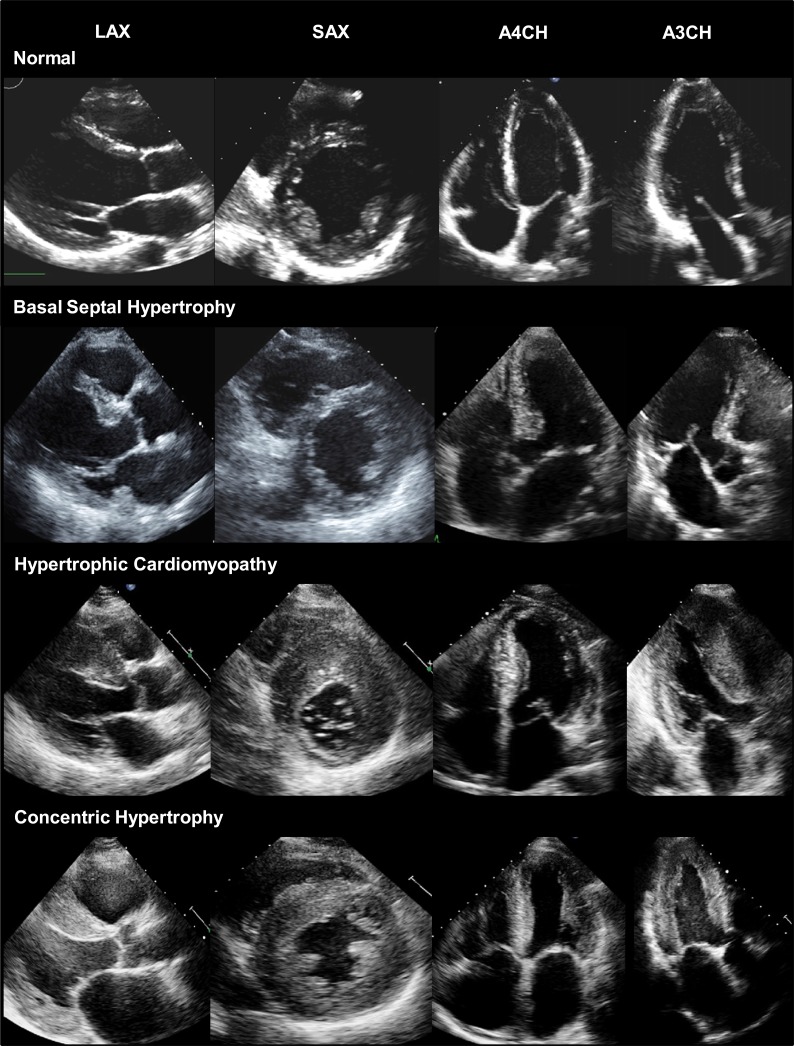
Comparison of normal myocardium, BSH, HCM, and concentric LVH on conventional two-dimensional echocardiography. PLAX = parasternal long axis view, SAX = short axis view, A4CH = apical 4-chamber view, A3CH= apical 3-chamber view.
